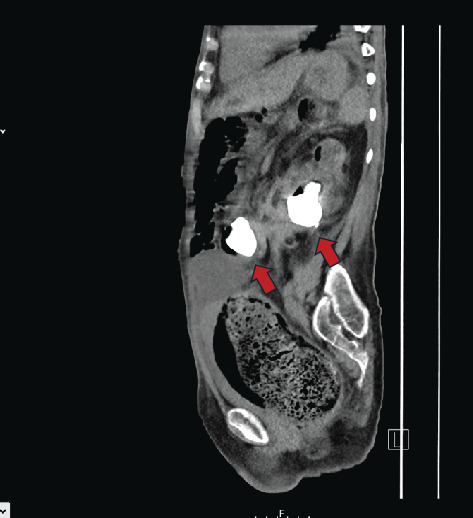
Initial CT scan, demonstrating the staghorn calculus within the renocolic fistula (left arrow) alongside the staghorn inside the left kidney (right arrow).
